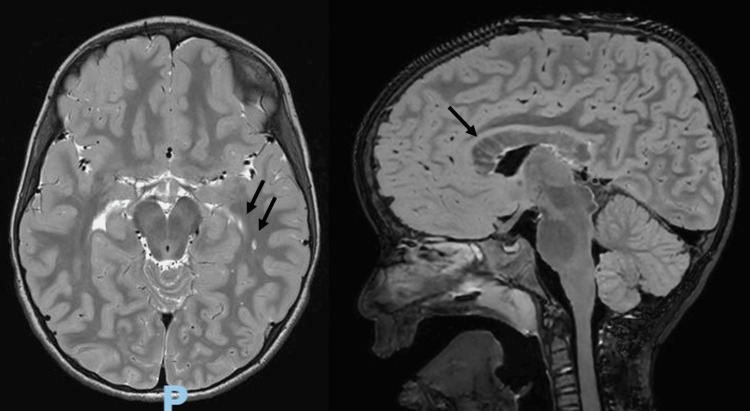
Enlarged perivascular spaces and callosal thickening on brain MRI Left panel: Brain MRI showing multiple enlarged perivascular spaces (Virchow-Robin spaces) involving the bilateral cerebral subcortical white matter and the entire length of the corpus callosum. Right panel: The corpus callosum is thickened, with the exception of the splenium, which is of normal thickness.
